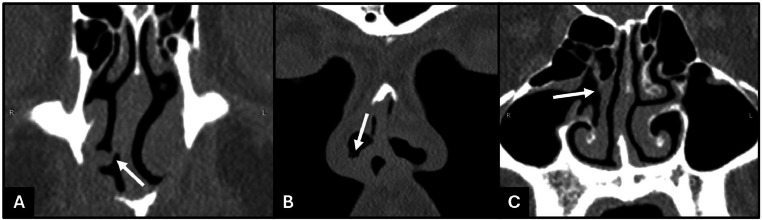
Coronal CT scans showed (A) the inferior part of the bifid inferior turbinate attached to the inferior nasal septum anteriorly, (B) forming a partition that divided the right nostril into 2 compartments, (C) and continued posteriorly to the mid-nasal septum and then attached to the inferior side of the right ethmoid sinus.
